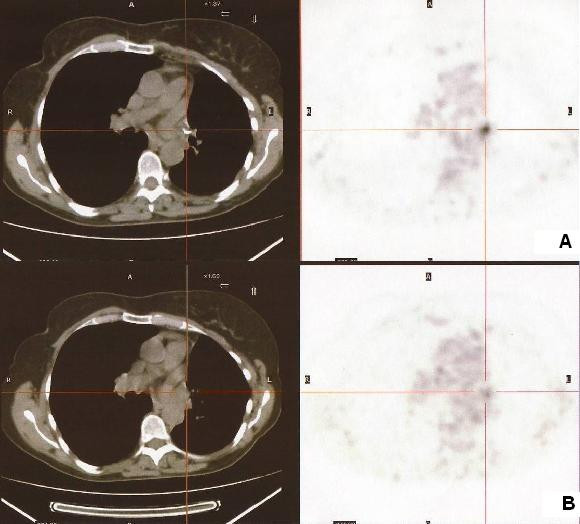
Fluorodeoxyglucose positron emission tomography and computed tomography scans before (A) and after (B) antifungal therapy.
